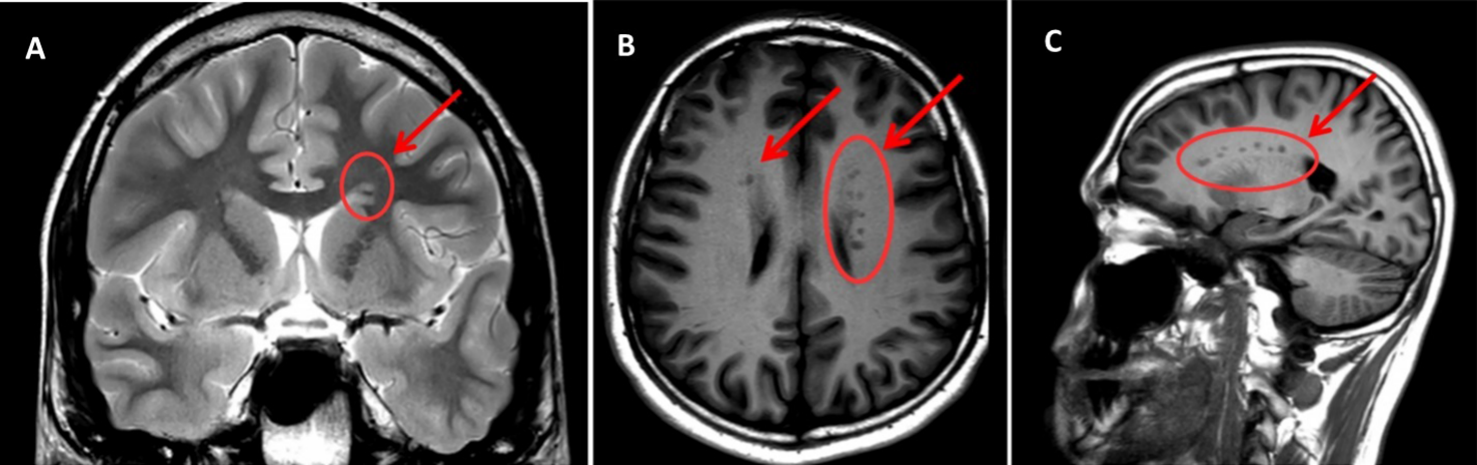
MRI scan sections of the patient's brain reporting the presence of GMH (indicated by arrows and circles). (A) T2W – coronal section; (B) T1W- assial section; (C) T1W – sagittal section. Arrows indicate areas of subependimal alterated signal (bilateral and asymmetrical PNH—Periventricular Nodular Heterotopia—with heterotopic grey matter stretching all along the ventricular walls, maximum diameter = 5 mm). Note that the shade of grey is the same as that of the cortical grey matter (the same signal intensity), which confirms that it is grey matter—the pathognomonic finding in GMH.
